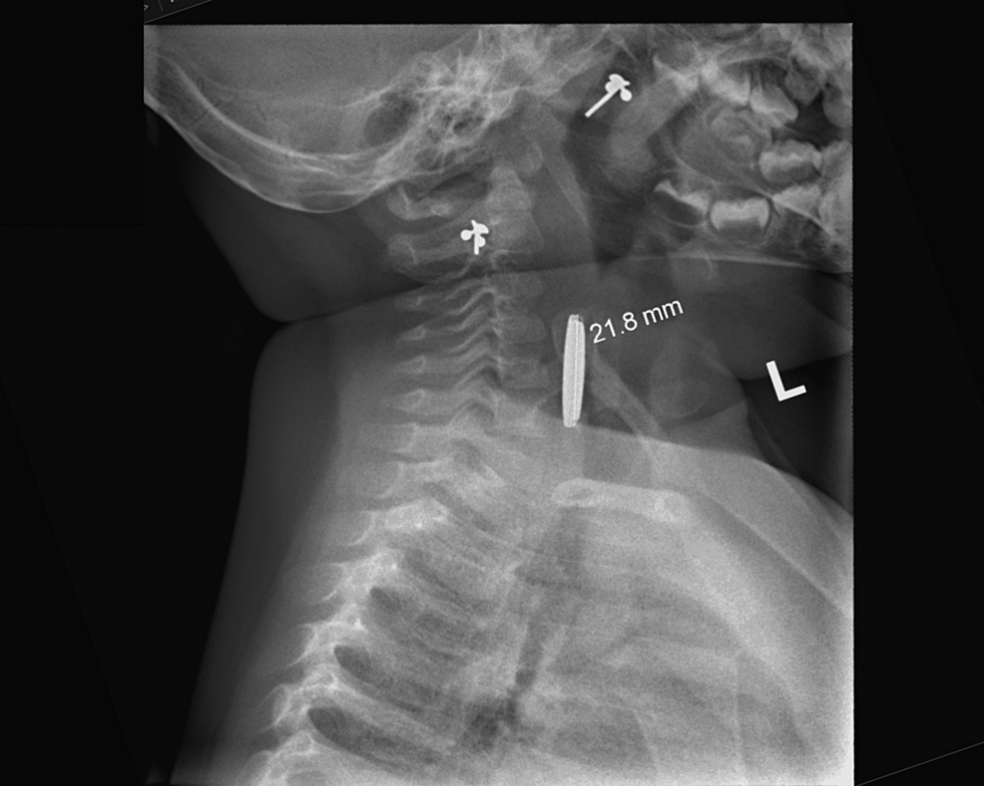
Lateral soft tissue neck X-ray
